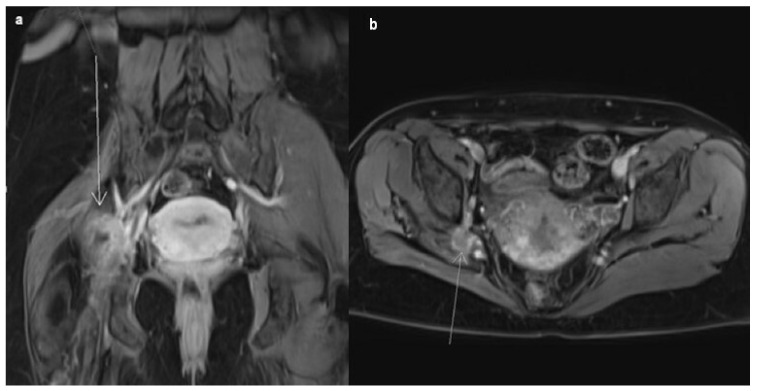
Postcontrast coronal (a) and axial (b) T1w FS MRI images present a heterogeneous mass in the right obturator fossa (white arrows), vaguely demarcated from the neurovascular pedicle. Note the large fibroids in the anterior uterine wall (picture (b)).
